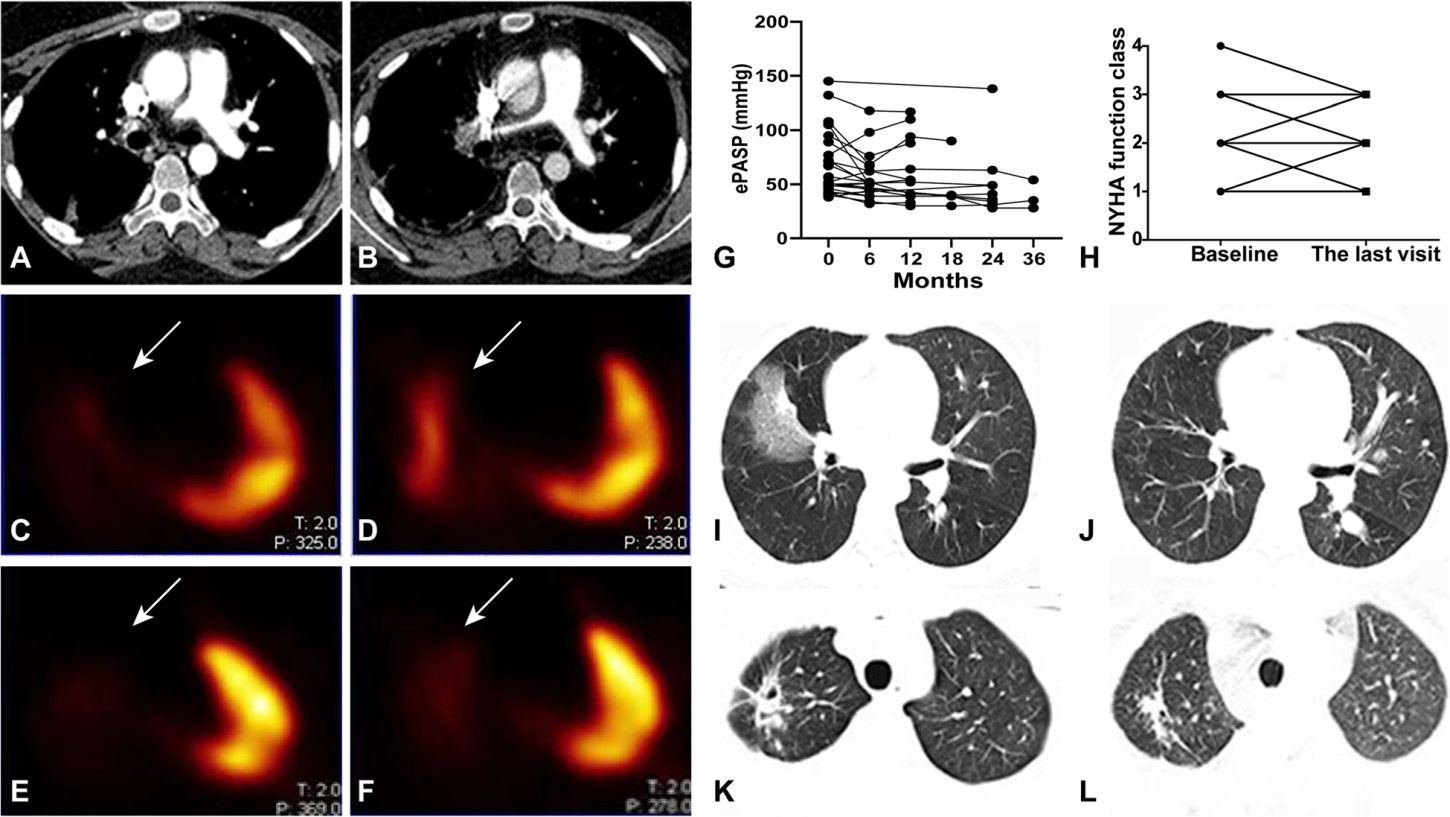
Pulmonary and cardiac conditions after treatment. a–f Pulmonary arterial changes before and after treatment: the right main pulmonary artery was shown upon CTA after balloon dilation intervention as well as immunosuppressive treatment in one patient (prednisone and azathioprine; a before treatment; b after treatment); blood supply to the right lung was also increased upon lung VQ scan after 1 year of treatment with prednisone and sirolimus in another patient (c, e before treatment; d, f after treatment). g The ePASP upon ultrasonography tended to decrease during patients’ follow-ups (baseline, 62.32 ± 28.76 mmHg, *n* = 27; 6th month, 58.73 ± 24.03 mmHg, *n* = 15; 12th month, 62.69 ± 29.75 mmHg, *n* = 13; 18th month, 49.75 ± 27.21 mmHg, *n* = 4; 24th month, 52.11 ± 34.01 mmHg, *n* = 9; 36th month, 39 ± 13.45 mmHg, *n* = 3) despite of treatment regimens. h Patients’ cardiac function was improved in most patients despite of treatment regimens. i, j Infarcted lesions dissipated after 12 months of treatment with prednisone and AZA as well as agents for PH in one patient. k, l The cavitation in the right lung apex became smaller after treatment with prednisone and sirolimus for 7 months in one patient
